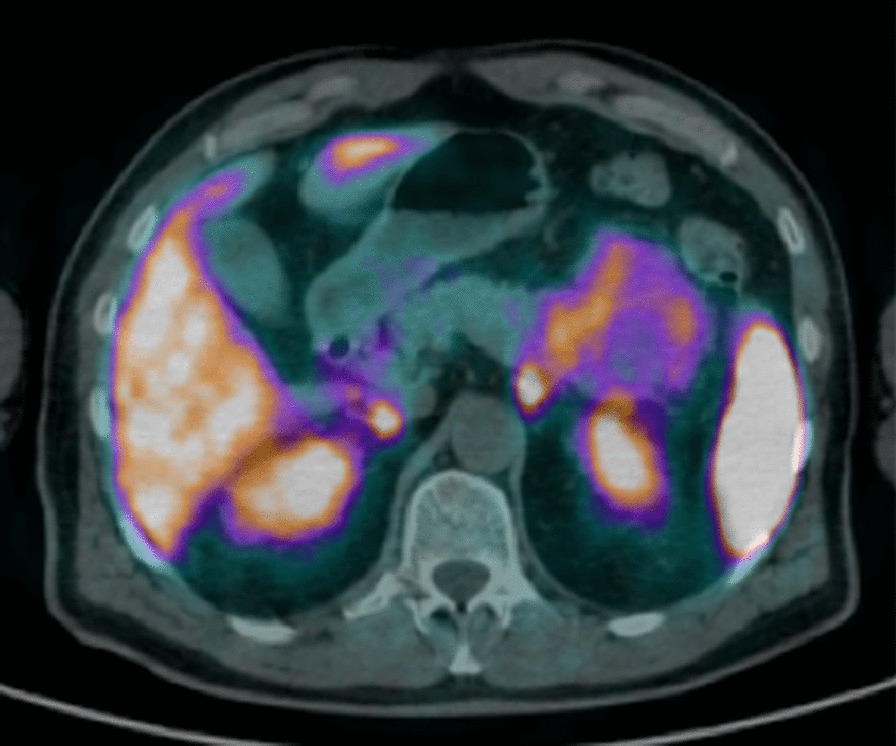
68Ga-DOTA-peptide (namely 68GaDOTATOC) PET-CT scan revealing a pathological uptake of the radiopharmaceutical in the pancreatic lesion
